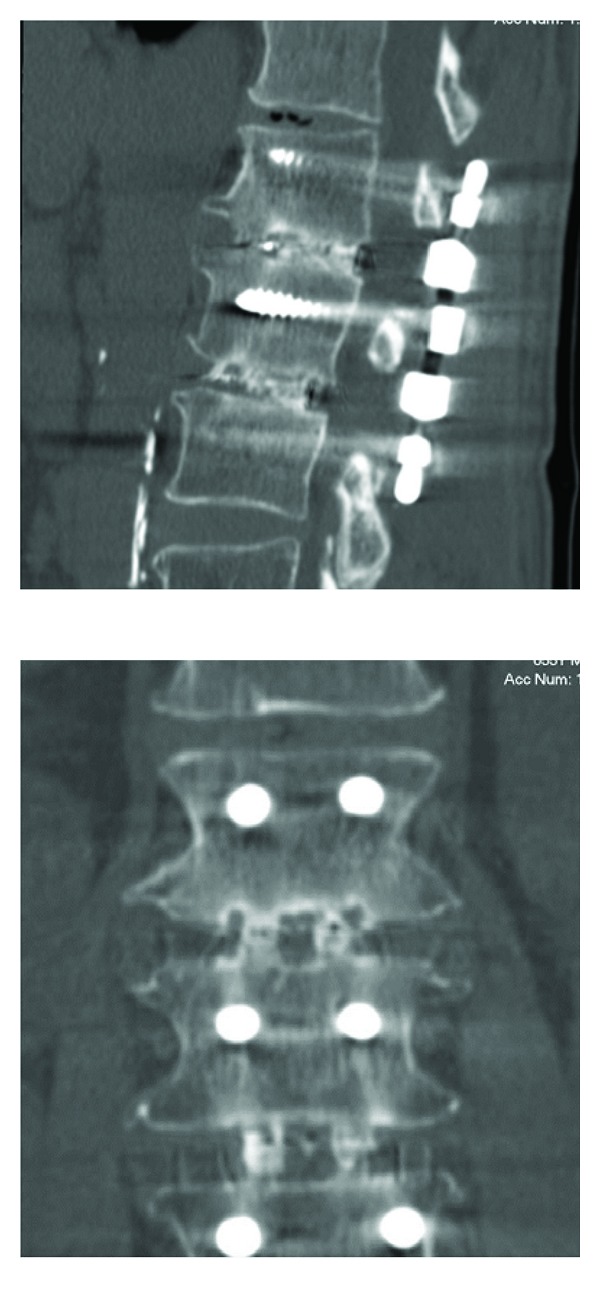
CT scan at 1-year FU showing solid fusion.
